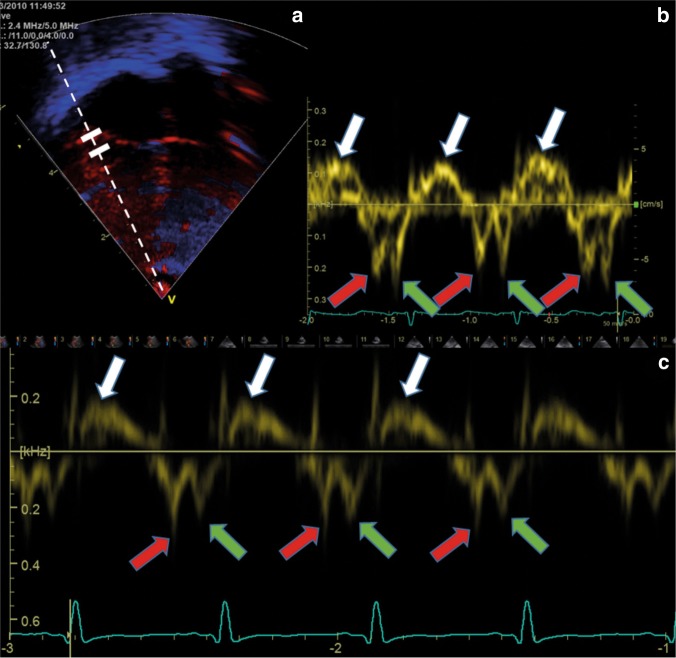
Pulsed-wave tissue Doppler velocity measurement from a term neonate, from the right lateral atrioventricular plane from the apical four-chamber view. The ultrasound system records the velocities from the area between the two calipers (the two white solid lines in the upper left panel). The dotted line denotes the direction of the ultrasound beams assessing the velocities. (Upper left panel) a Color-coded tissue Doppler image. (Upper right panel) b Spectral velocity time curve of three heart cycles, with relatively high gain setting. The upper panels (a and b) show a relatively poor image quality (blurred 2D image (a) and a broad spectral velocity–time curve (b). The lower panel c shows a velocity curve with narrower spectral curve, indicating better quality. *X*-axis: time. *Y*-axis: tissue velocity from the sample area. White arrows at the peak systolic velocities (s′), red arrows at the early diastolic velocities (e′), and green arrows at the peak velocity in atrial systole (a′)
